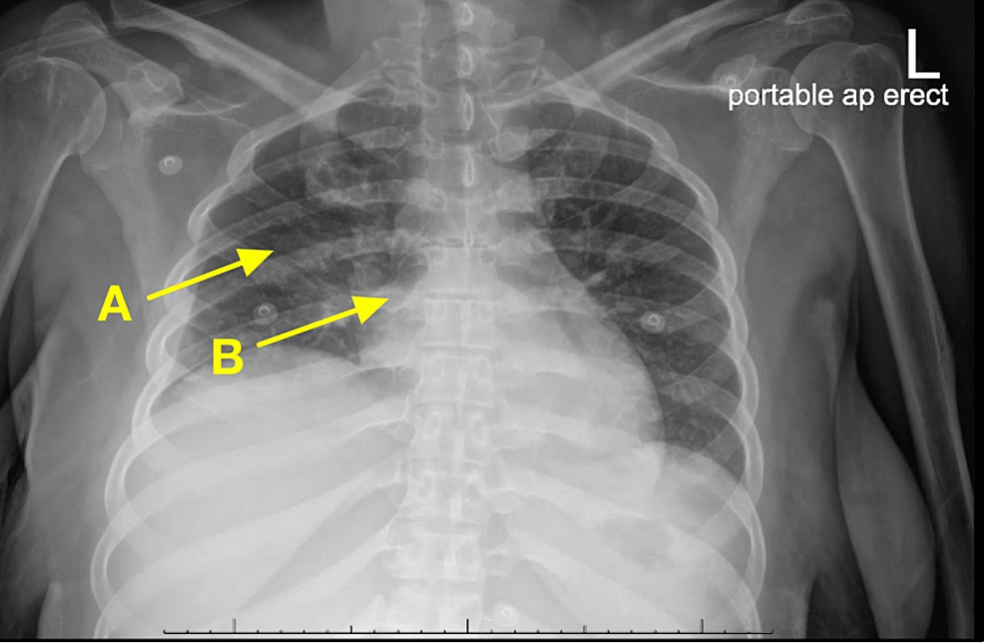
Chest X-ray revealing (A) hypoventilation with (B) resultant crowding of the pulmonary vasculature.
